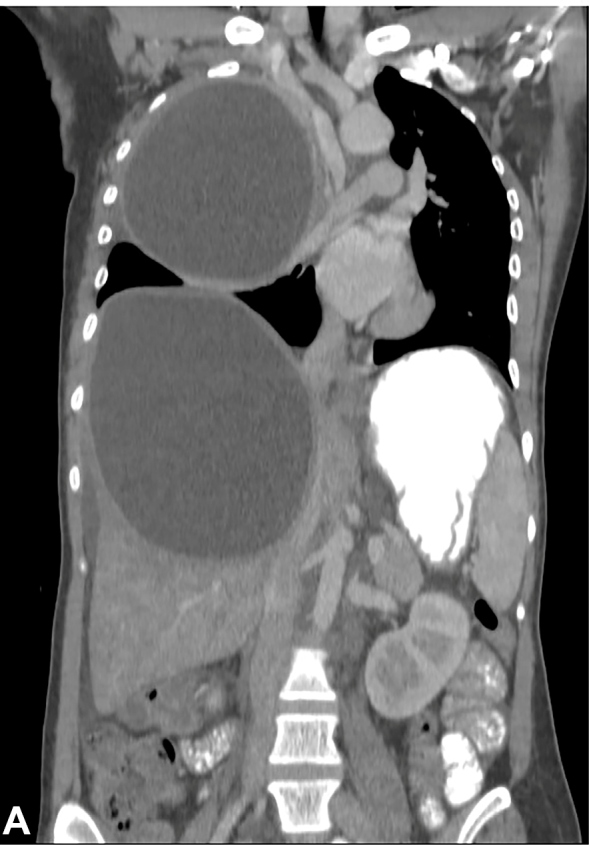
Computed tomographic scan of the abdomen showing one cyst (15 × 14 cm) in the right lobe of the liver.
